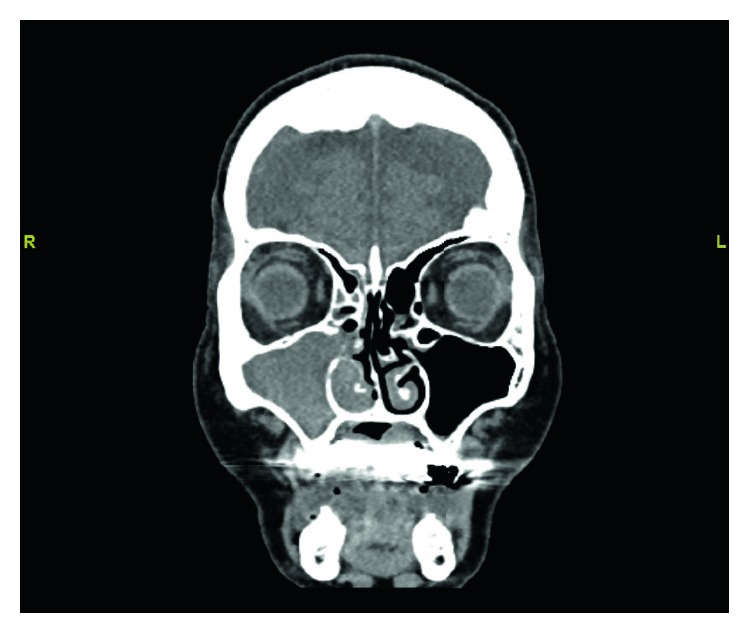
Coronal CT scan, soft tissue window, showing complete opacification of the right maxillary sinus, obstruction of the right ostiomeatal complex, and soft tissue density in the right inferior meatus.
